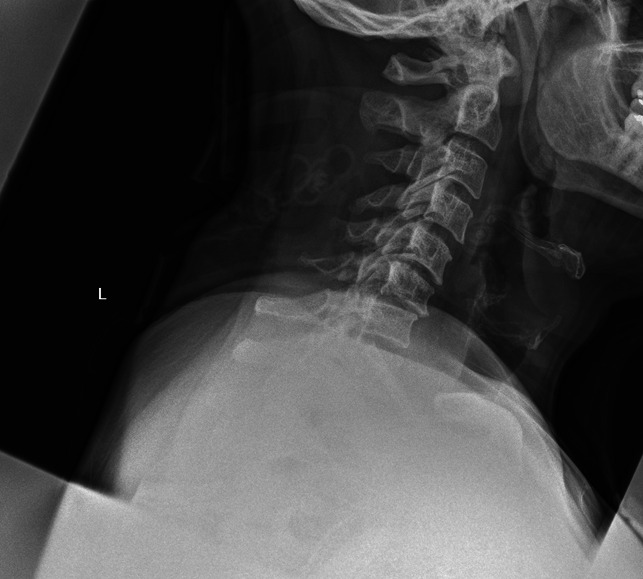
Erect c-spine radiograph conducted on arrival to clinic showing instability.
